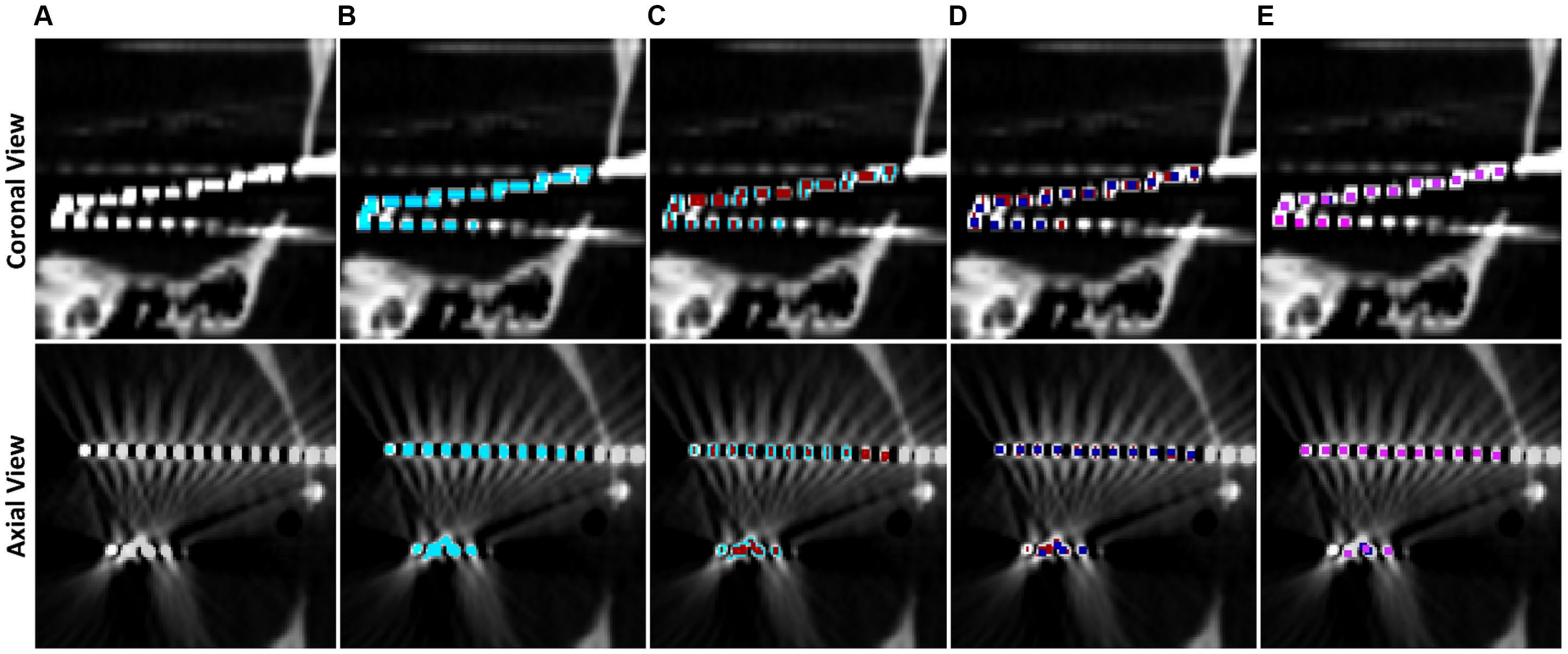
Step by step example of electrode contact segmentation and representative replacement. The top row is a cropped coronal slice while the bottom row is a cropped axial slice. (A) Base CT image, note that the ends of two electrodes have blended on the imaging and there is streaking artifact connecting two contacts. (B) Post masking, filtered, and thresholded electrode contact mask overlaid in blue. (C) Electrode contact mask after first pass of erosion in red, note that the ends of the electrodes have separated. (D) Second pass of erosion in dark blue. Contacts connected by streaking artifact have separated. (E) Final pass of the algorithm in pink. All contacts have been replaced by the representative contact by now.
